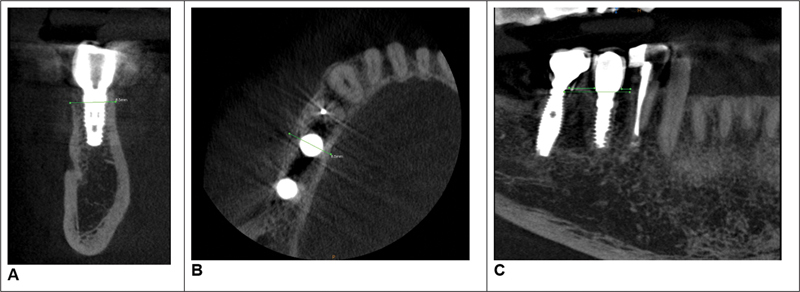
Cone-beam computed tomography (CBCT) images of patient 1, after 1 year.
